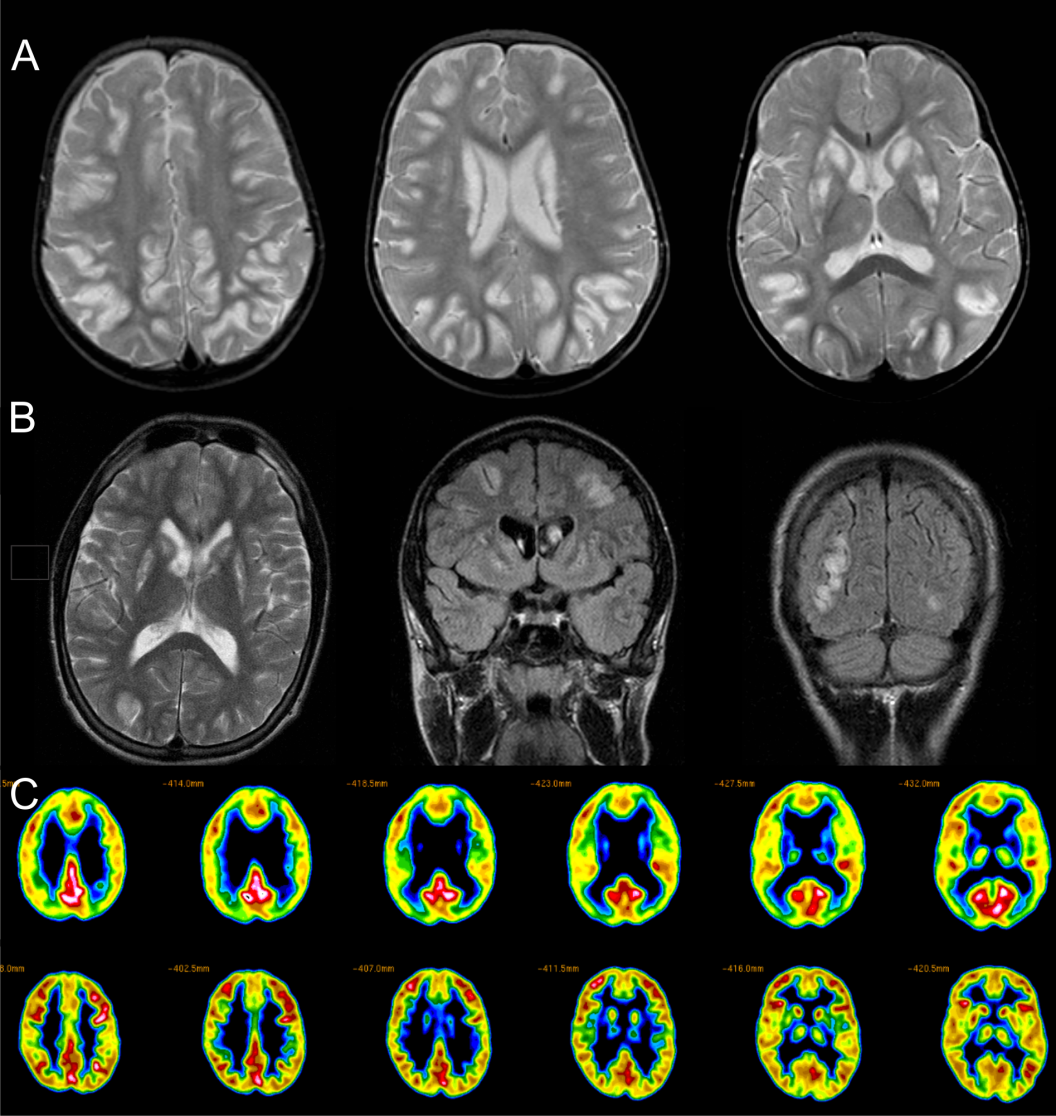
Neuroimaging findings. A: axial T2 weighted MRI of patient 2 taken during an episode with seizures, increasing encephalopathy and exacerbation of the dystonia. Images show bilateral high T2-signal changes and swelling in the putamen and caudate head. In addition there are multiple cortical lesions preferentially occurring in the depths of sulci, which is typical of BBGD. B: axial T2 and coronal T2-FLAIR weighted MRI of patient 1 taken during an episodic exacerbation show a similar pattern with bilateral striatal and multiple cortical lesions. The signal abnormalities regressed completely on later scans (not shown). C (upper lane): FDG-PET scan of the brain of patient 1 taken ~5 years later shows multiple foci of decreased glucose metabolism that correlate to the localization of the transient cortical signal changes on MRI. The striatum shows minimal uptake consistent with severe neuronal loss. A normal scan is shown in the lower lane for comparison.
